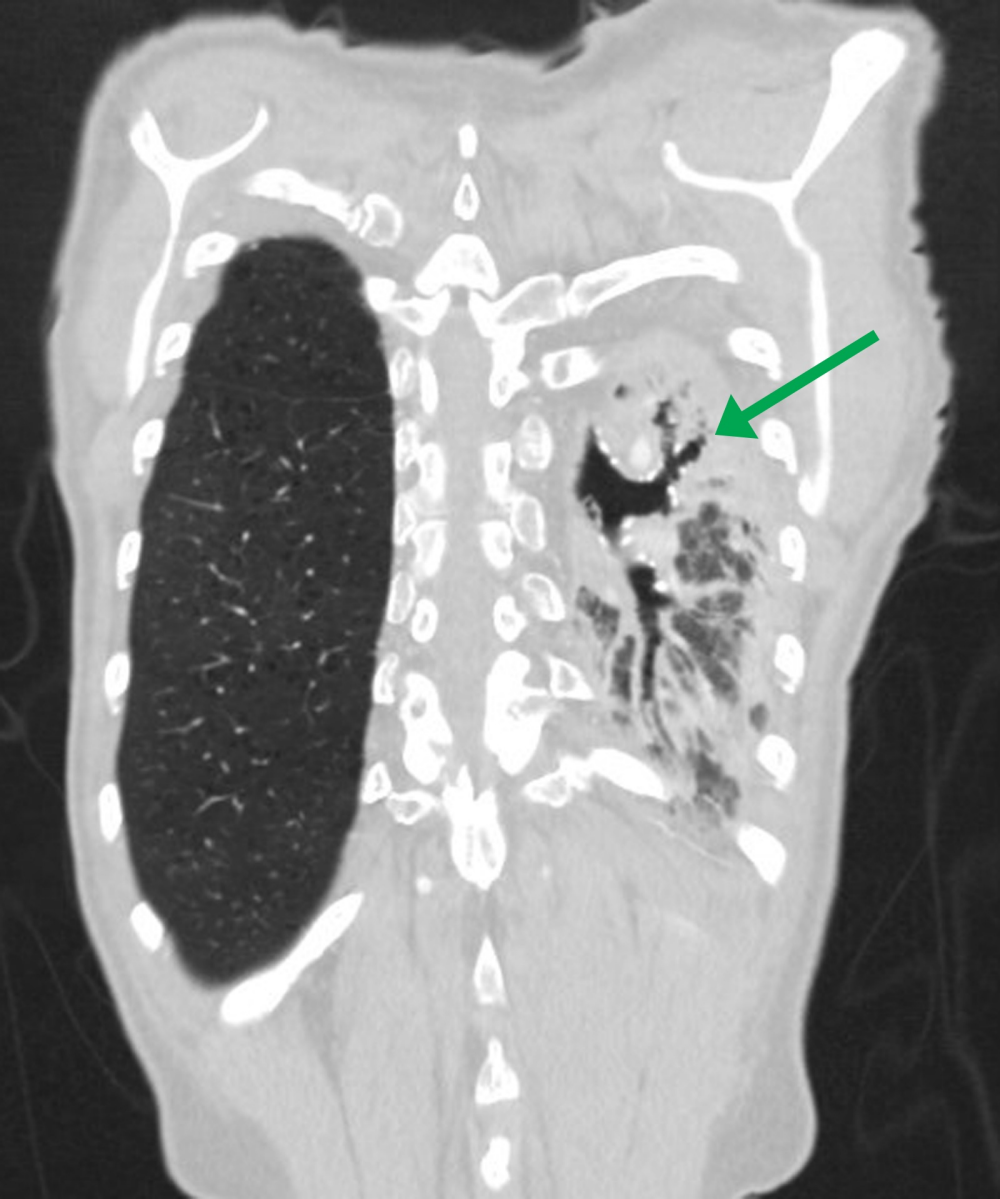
Contrast-enhanced CT chest, coronal reformation in lung window. Bronchiectasis may be seen in cases of pulmonary artery agenesis secondary to recurrent pulmonary infections (green arrow).
